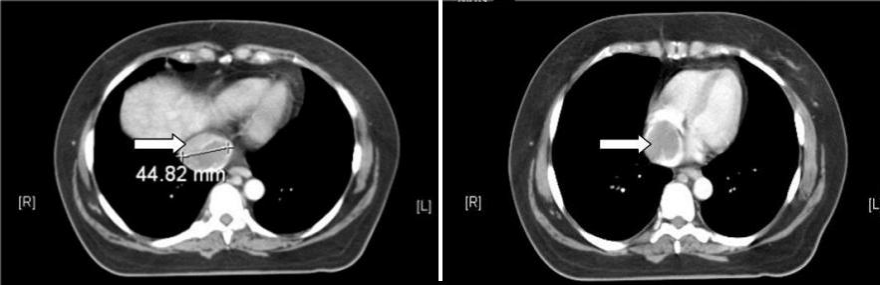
CT Abdomen showing leiomyosarcoma in the inferior vena cava. On the left (a), note that the transverse diameter of the mass is approximately 4.5 cm. On the right (b), the tumor mass is seen clearly within the right atrium.
